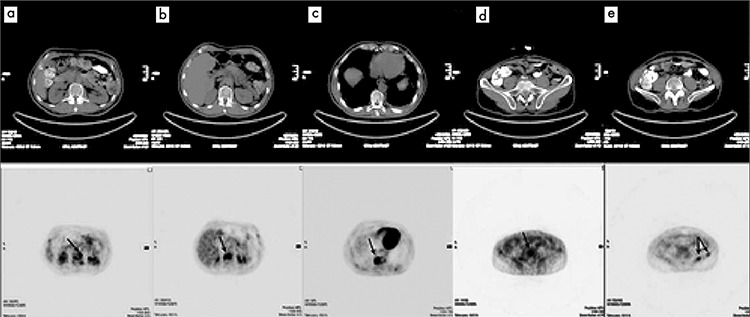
Selected axial slices of PET and CT images from F 18 FDG PET/CT scan showing pathologically increased FDG uptake at dorsal vertebrae (a, b, c), fifth lumber vertebra (d) and left iliac wing (e) without any corresponding lytic or sclerotic lesion on CT images
